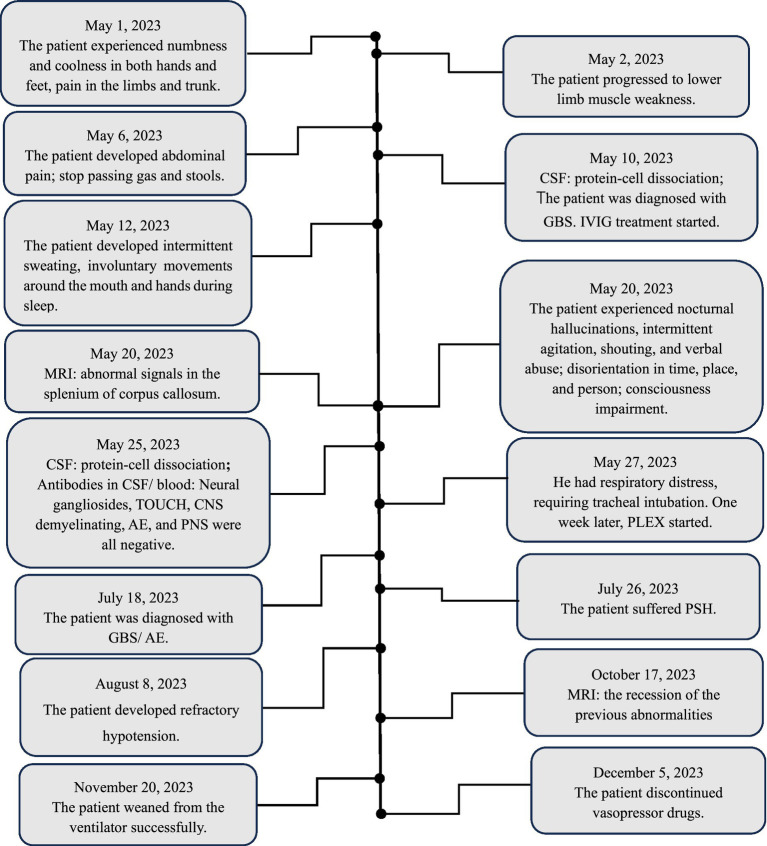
Patient timeline. CSF: cerebrospinal fluid; WBC: white blood cell; CNS: central nervous system; PNS: paraneoplastic syndrome; GBS: Guillain-Barré syndrome; IVIG: intravenous immunoglobulin; MRI: magnetic resonance imaging; AE: autoimmune encephalitis; PLEX: plasma exchange; PSH: paroxysmal sympathetic hyperactivity.
